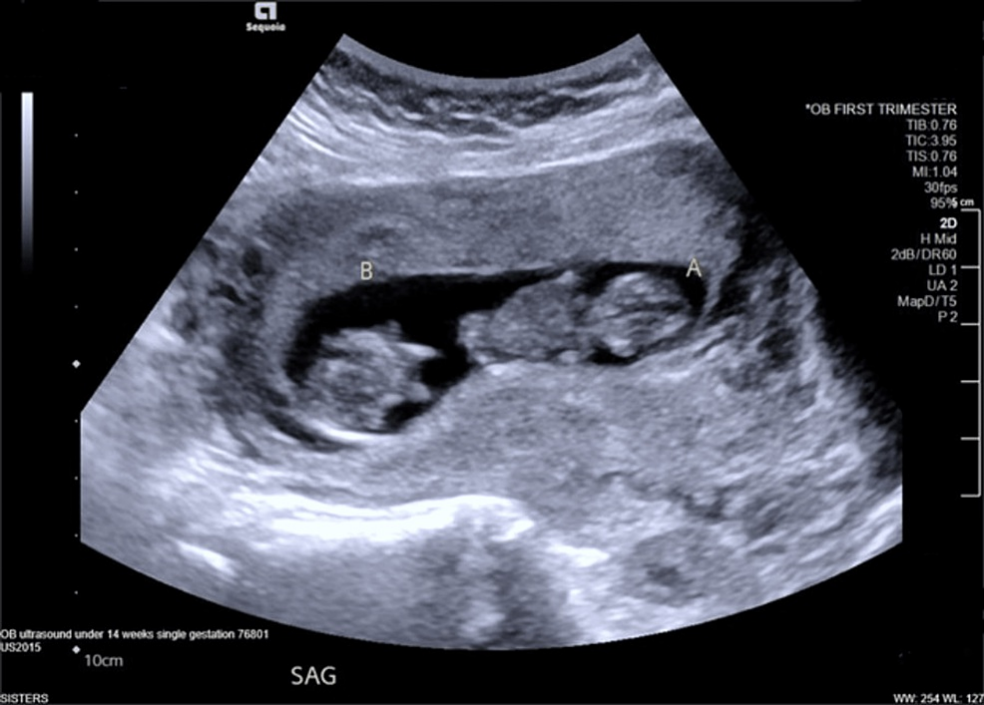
Dating ultrasound at 11 weeks showing twin intrauterine gestation without an apparent dividing membrane
